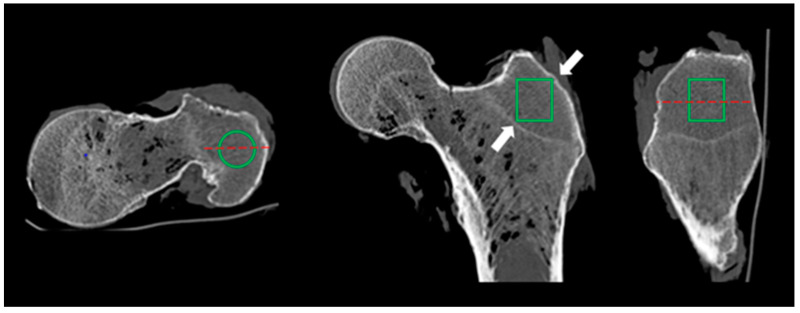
Example of great trochanteric CT cylindric VOI. The VOI is placed from top to bottom in the middle of a line joining external cortical bone and physis line and a line joining anterior and posterior cortex (red dashed lines). The placement avoided the inclusion of cortical bone and physis line in the VOI (white arrows).
